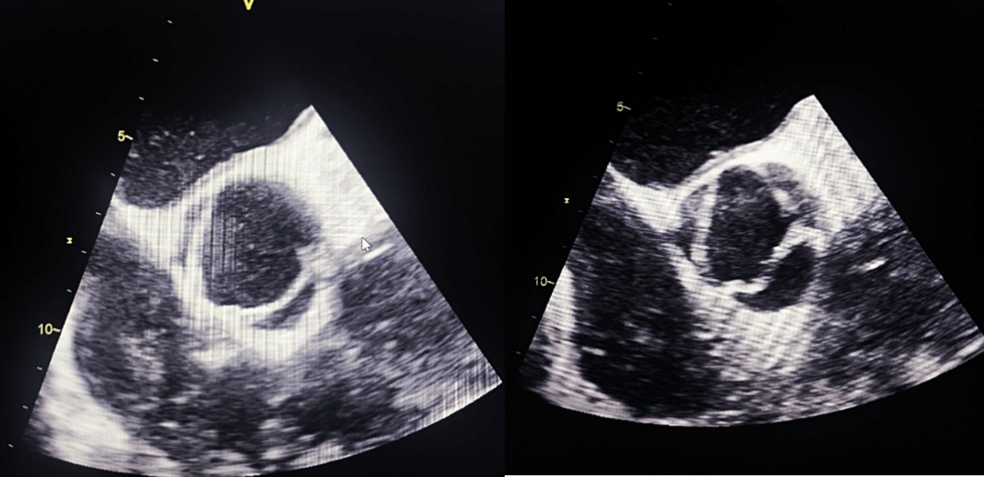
Transesophageal echocardiography showing left-right type 1 bicuspid aortic valve.
